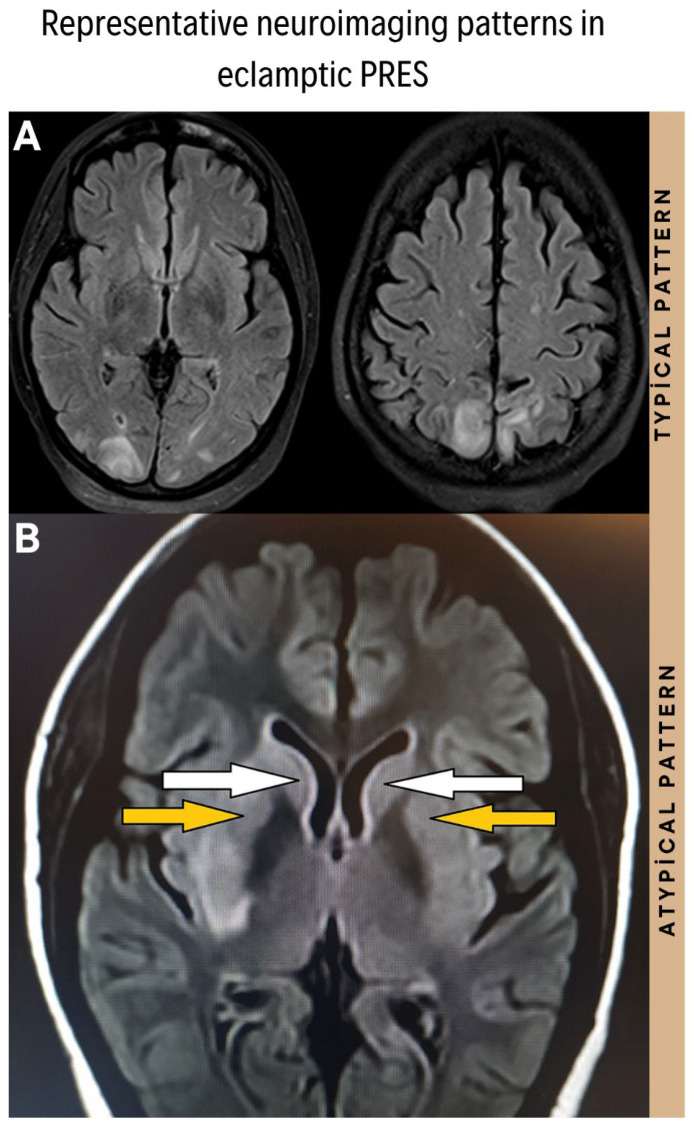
Representative neuroimaging patterns (Axial FLAIR MRI) in patients with eclampsia. (A) Typical PRES Pattern (Upper Panel): Axial FLAIR image reveals characteristic symmetrical hyperintense signals (vasogenic edema) primarily localized in the parieto-occipital lobes. (B) Atypical PRES Pattern (Lower Panel): Axial FLAIR image demonstrates extensive involvement of non-canonical regions, including the deep gray matter (thalamus and basal ganglia), representing a high-burden phenotype. White arrows indicate hyperintensities in the head of the caudate nucleus bilaterally, and yellow arrows indicate hyperintensities in the putamen region bilaterally.
